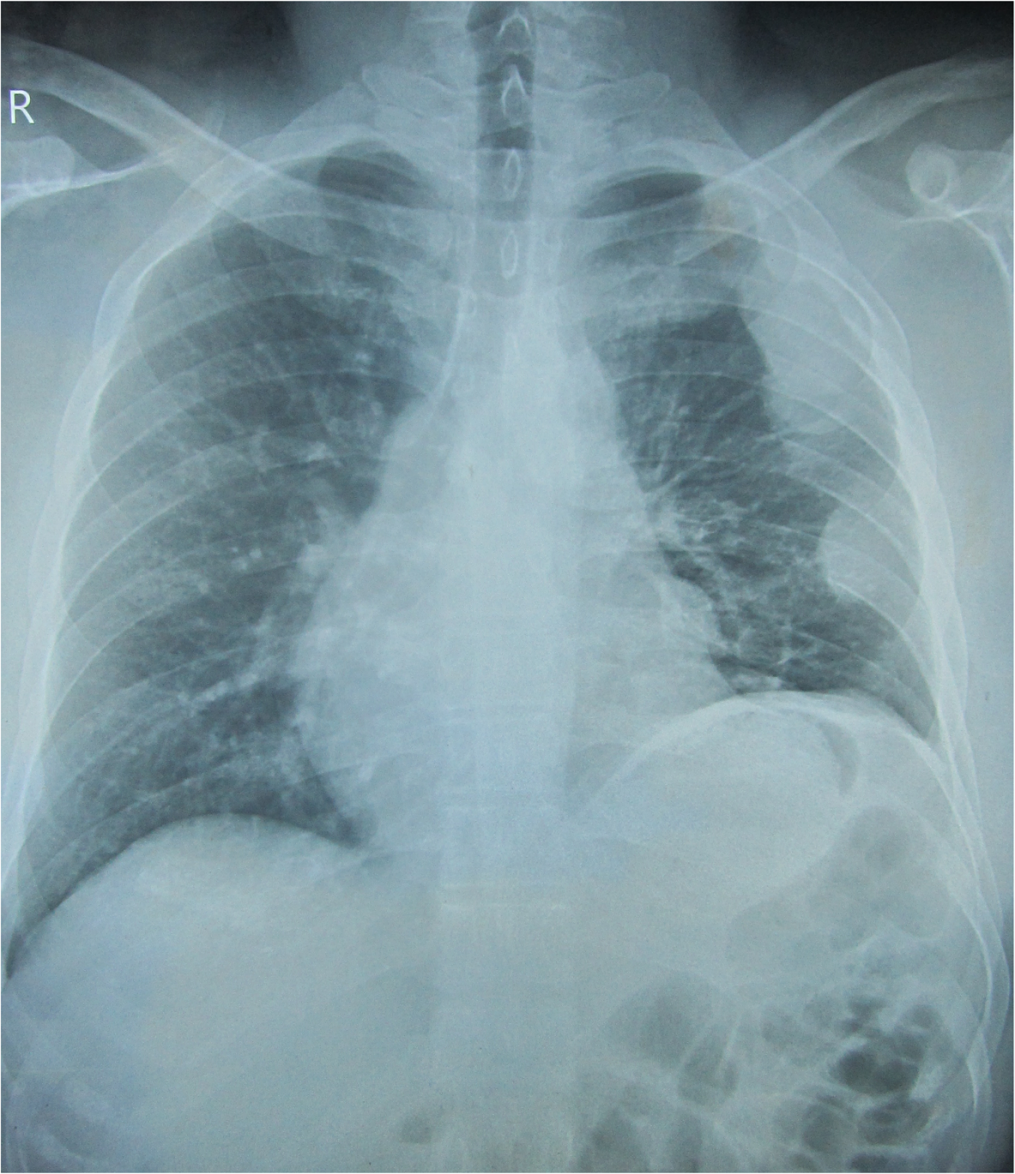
Chest X-ray posteroanterior view showing pleural-based deposits on the left side
